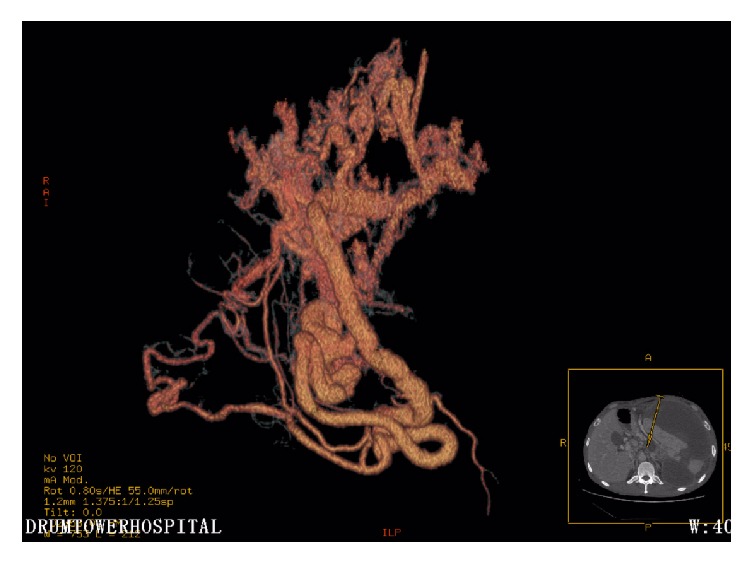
Vascular restructure with CT showing splenic vein dilation and expansion of portal vein branches.
